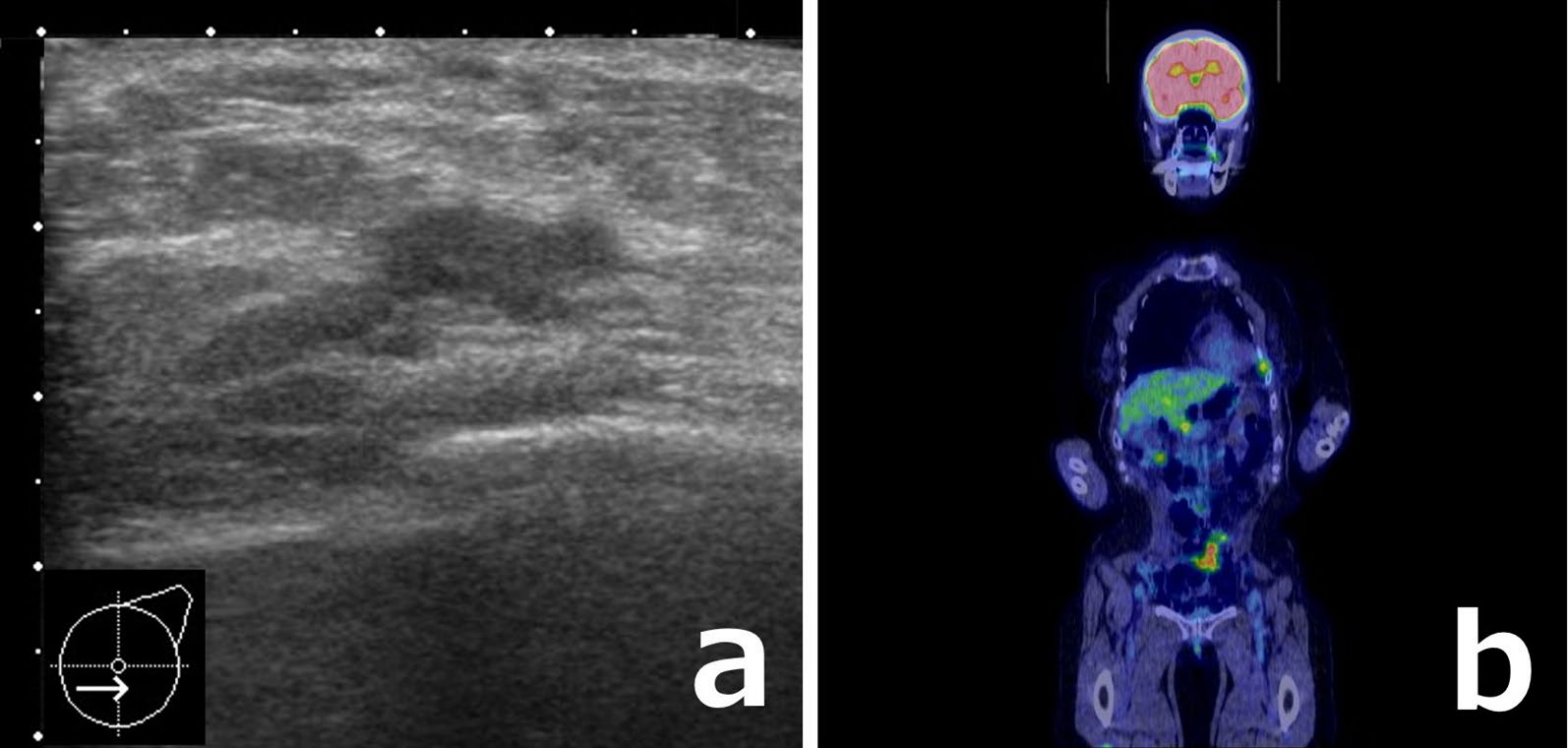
Case 2: imaging findings. a Ultrasound revealed a low-echo area of 18 × 6 × 13 mm with an unclear boundary in the lower-inner quadrant of the left breast. b Positron emission tomography–computed tomography showed abnormal accumulation in the rectal excision, peritoneal thickening in the pelvis, and other nodules in the abdominal cavity
